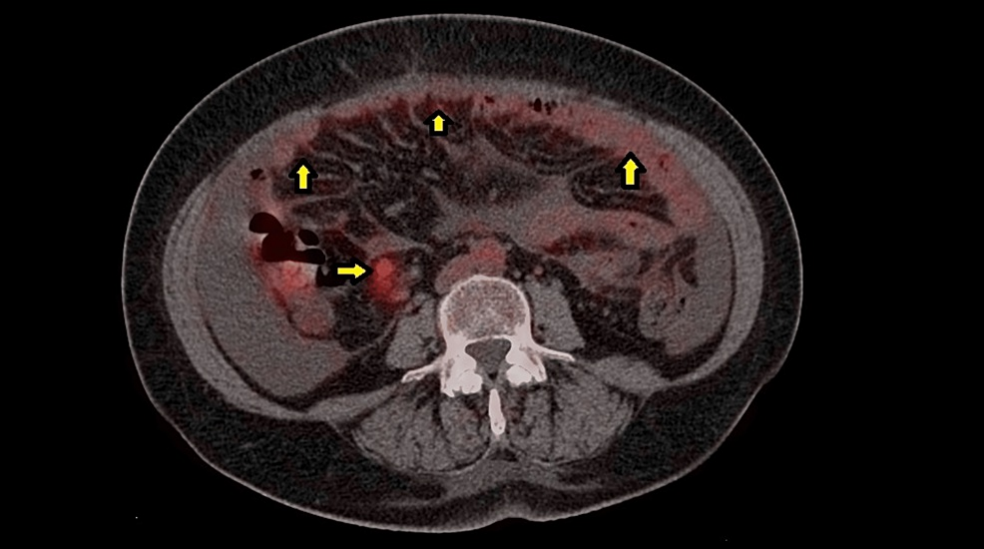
FDG avid lymph nodes and multiple areas of high uptake of the peritoneum (yellow arrows) noted on the PET scan. FDG: fluorodeoxyglucose, PET: positron emission tomography
